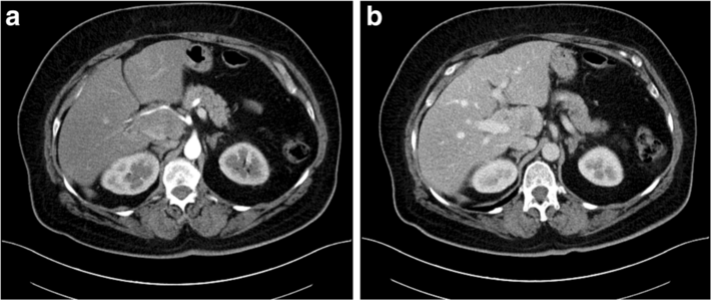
The preoperative contrast enhanced computed tomography scan demonstrated a heterogenous mass in the liver hilum which infiltrated the right portal vein. The hepatic artery was displaced by the tumor. However, CT did not reveal infiltration of the hepatic artery
